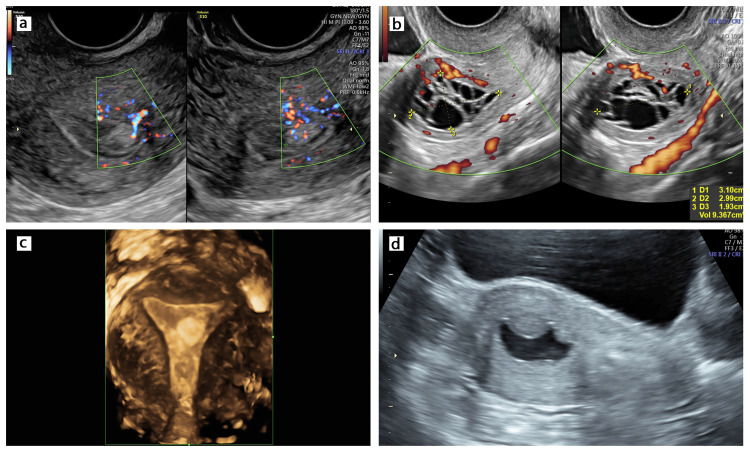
Ultrasound images of endometrial polyps. (a) Hyperechoic lesion with a feeder vessel on Doppler. (b) Polyp in a postmenopausal woman showing multiple cystic spaces. (c) 3D image of a polyp. (d) Polyp at saline sonography
